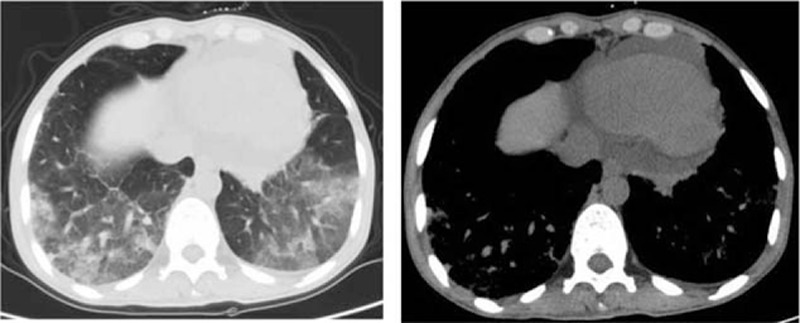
Chest computed tomography showing bilateral extensive consolidation at peripheral areas.
